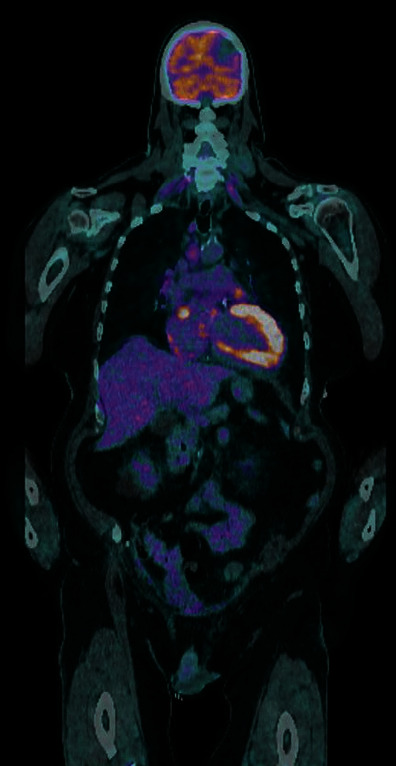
Coronal cardiac positron emission tomography (PET) image demonstrating absence of FDG avidity at the bioprosthetic aortic valve. Physiological FDG avidity is noted throughout the myocardium of the left ventricular wall.
